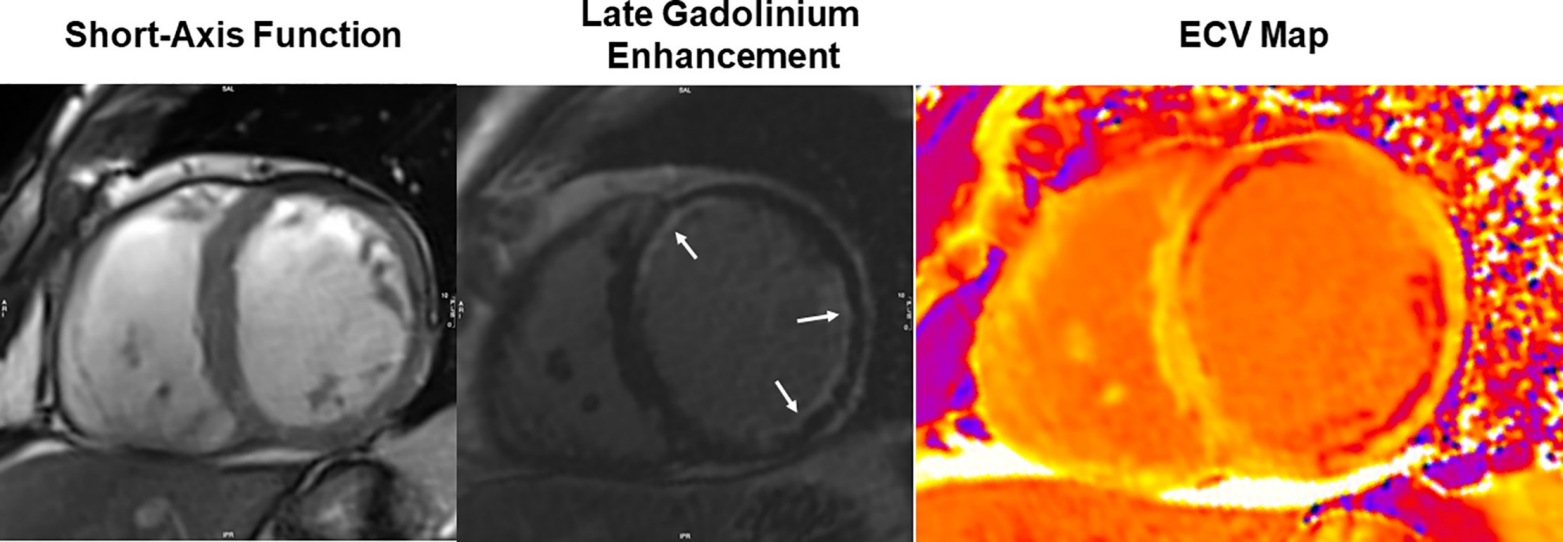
Quantitative assessment of cardiac remodeling and myocardial fibrosis by cardiac magnetic resonance imaging. From left to right, a short-axis cine image, a late gadolinium enhancement (LGE) infarction image, and a myocardial extracellular volume (ECV) map in a matching short-axis location of the left ventricle are shown, respectively. LGE characterizes areas of subendocardial infarct (white arrows). An ECV map was constructed by serial T1 maps before and after gadolinium contrast injection. Non-infarct fibrosis was determined from the ECV maps with matching segments containing LGE removed.
